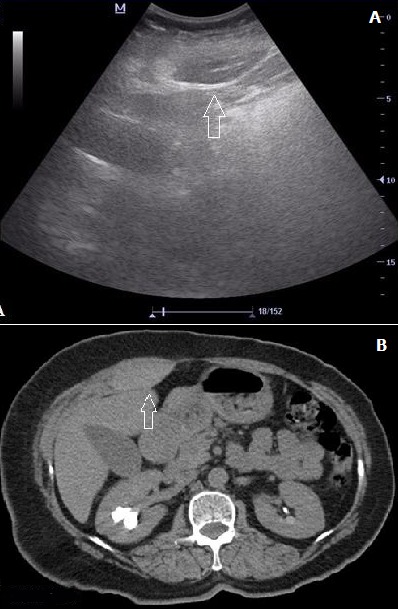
Bedside ultrasonographic view of the cystic mass in the anterior abdominal wall; (B) enhanced CT scan showing a 5.8x2.7 cm ovoid dilatation in the right rectus muscle and thickening of the abdominal wall muscles adjacent to the rectus
